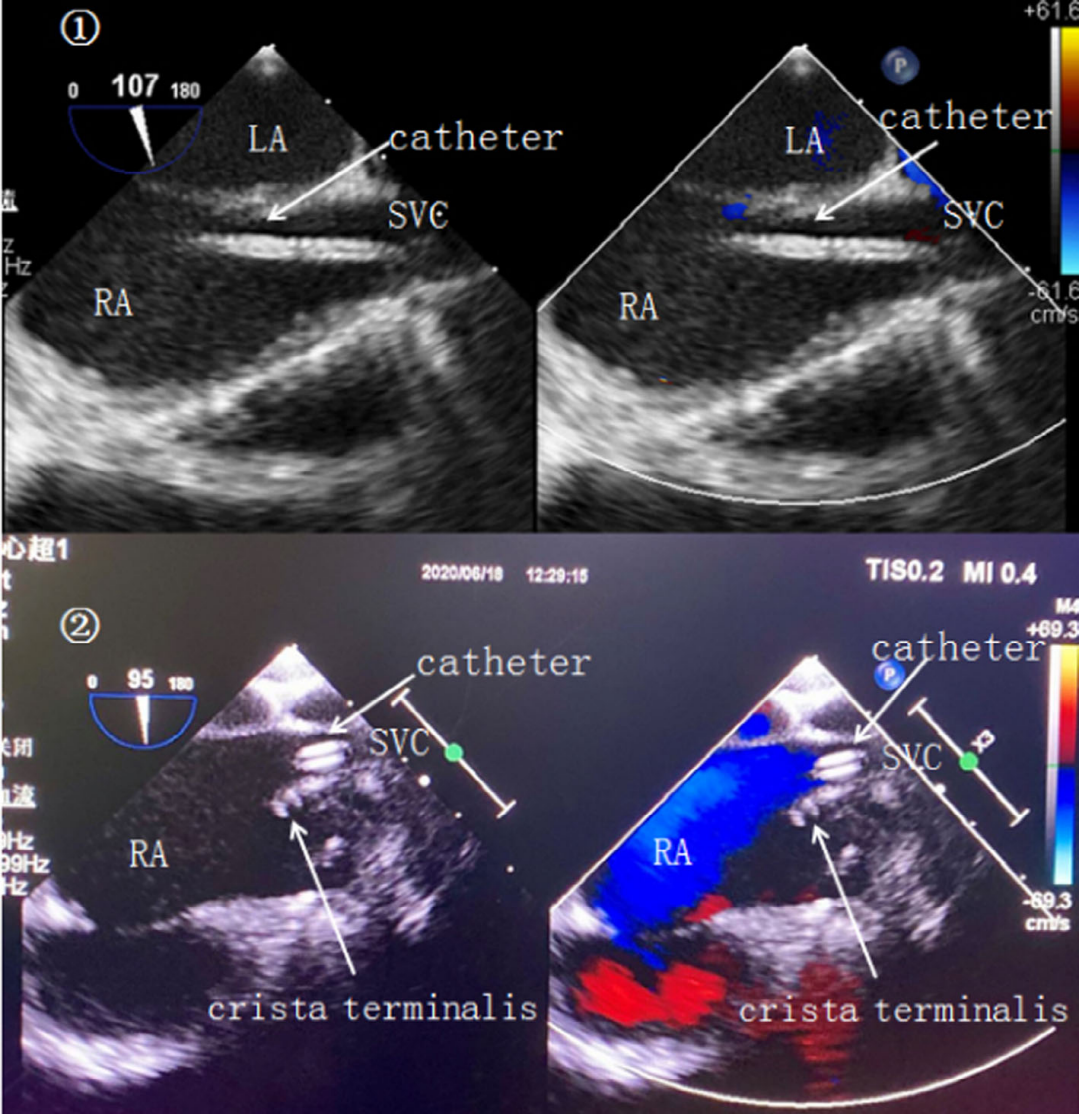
TEE images during catheter puncture and localization. (A) TEE image of the catheter entering the SVC inlet during the left subclavian vein puncture. (B) TEE image of the catheter tip finally positioned above the crista terminalis. RA, right atrium; SVC, superior vena cava; TEE, transesophageal echocardiography
